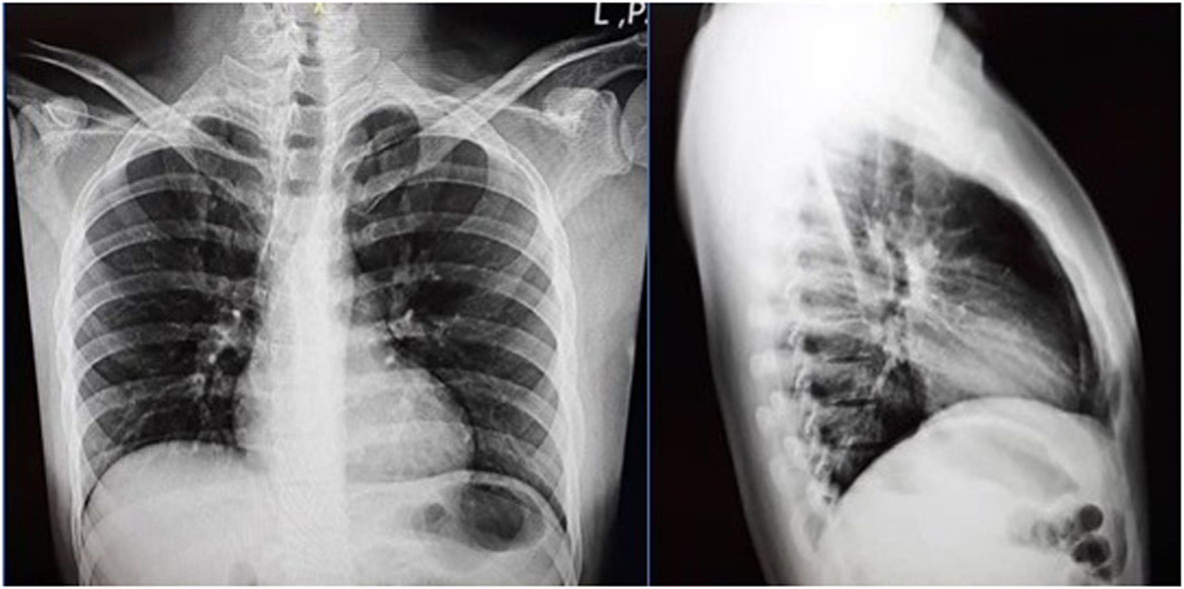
Chest radiography did not show any abnormalities
